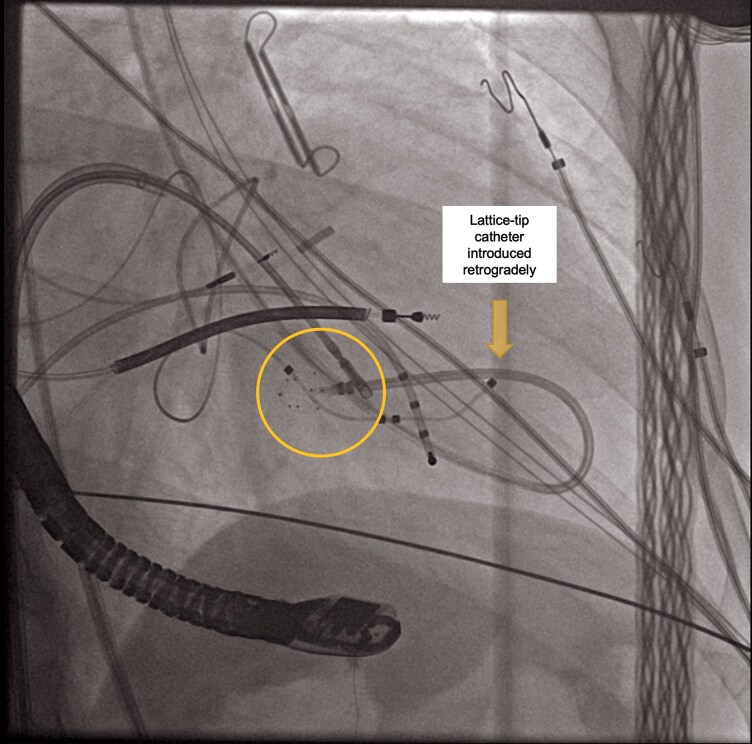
Fluoroscopic image (right anterior oblique view) depicting retrieval of the lattice-tip catheter entrapped within the neochordal apparatus. A bioptome, inserted through a steerable sheath, is advanced towards the lattice-tip catheter, which is caught between the chordae tendineae and the posteromedial papillary muscle. The bioptome jaws (highlighted by the circle) grasp the lattice-tip catheter to facilitate controlled extraction after unsuccessful traction attempts under echocardiography guidance.
